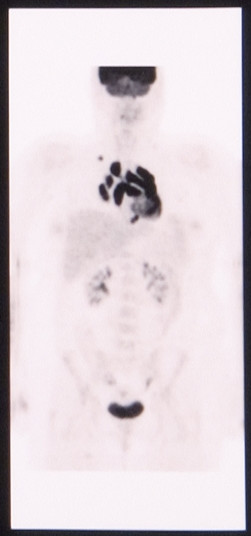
PET/CT showing extensive hypermetabolic mediastinal adenopathy.
